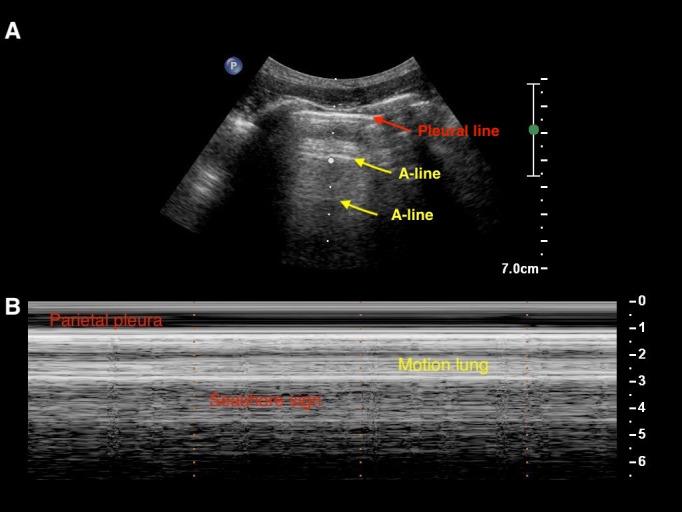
Basic signs of lung ultrasound in B- and M-mode, normal lung. (A) In B-mode, the red arrow indicates the pleural line (horizontal echogenic line under subcutaneous tissue). The pleural line can be observed moving with respiratory movement. The yellow arrows represents the horizontal A-lines. (B) The M-mode demonstrates normal pleura sliding: subcutaneous tissues above the pleural line do not move away or toward the probe and are represented as straight lines. The pattern below the pleura is an artifact deriving from visceral pleura sliding, as it generates a sandy pattern called the “seashore sign.”
